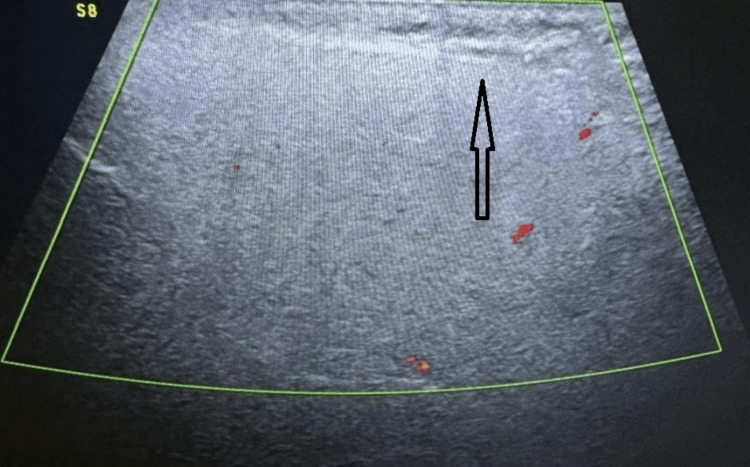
Ultrasonography of the swollen right parotid gland Black arrow represents swelling in the right parotid gland
